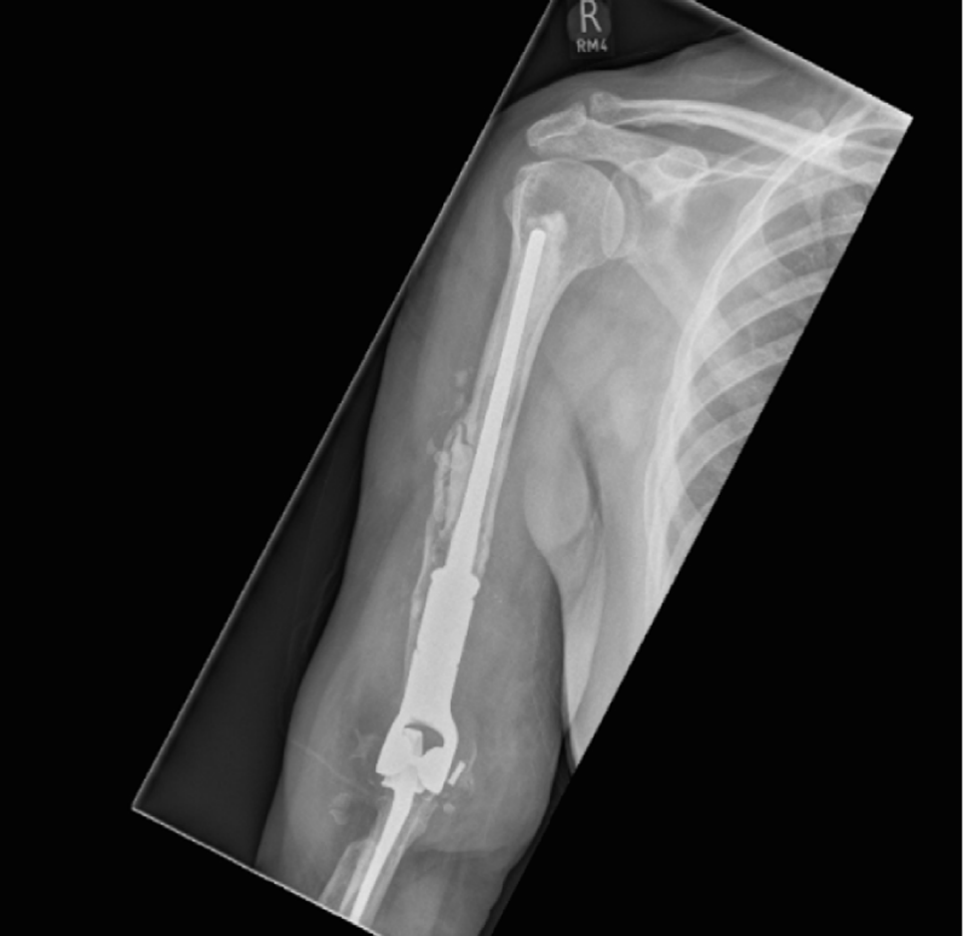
This figure shows the pre-operative radiograph for the second case, which also shows significant loosening around the endo-prosthetic stem with a peri-prosthetic fracture and a broken elbow prosthetic hinge.
